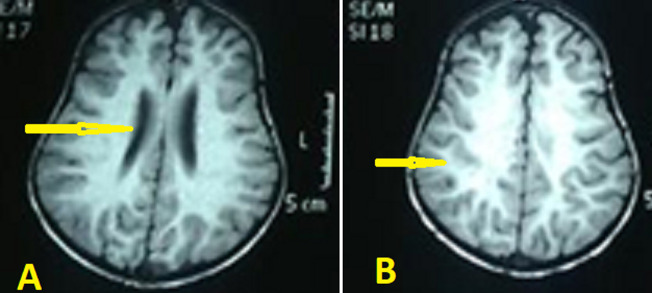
brain magnetic resonance imaging showing A) mild dilated third ventricle (arrow); B) prominent cortical sulci and sylvan fissures (arrow)
